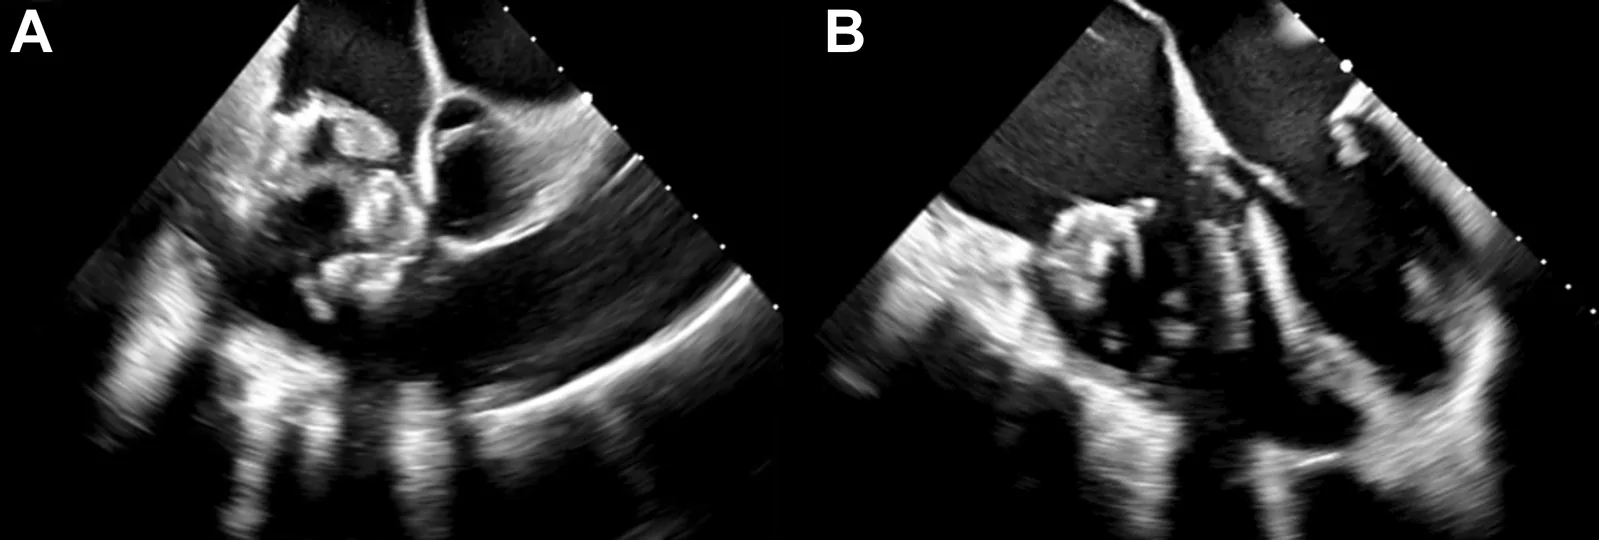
Transesophageal Echocardiography Shows Valve Embolization in 2 Views (A and B) Transesophageal echocardiography shows valve embolization in 2 views.
